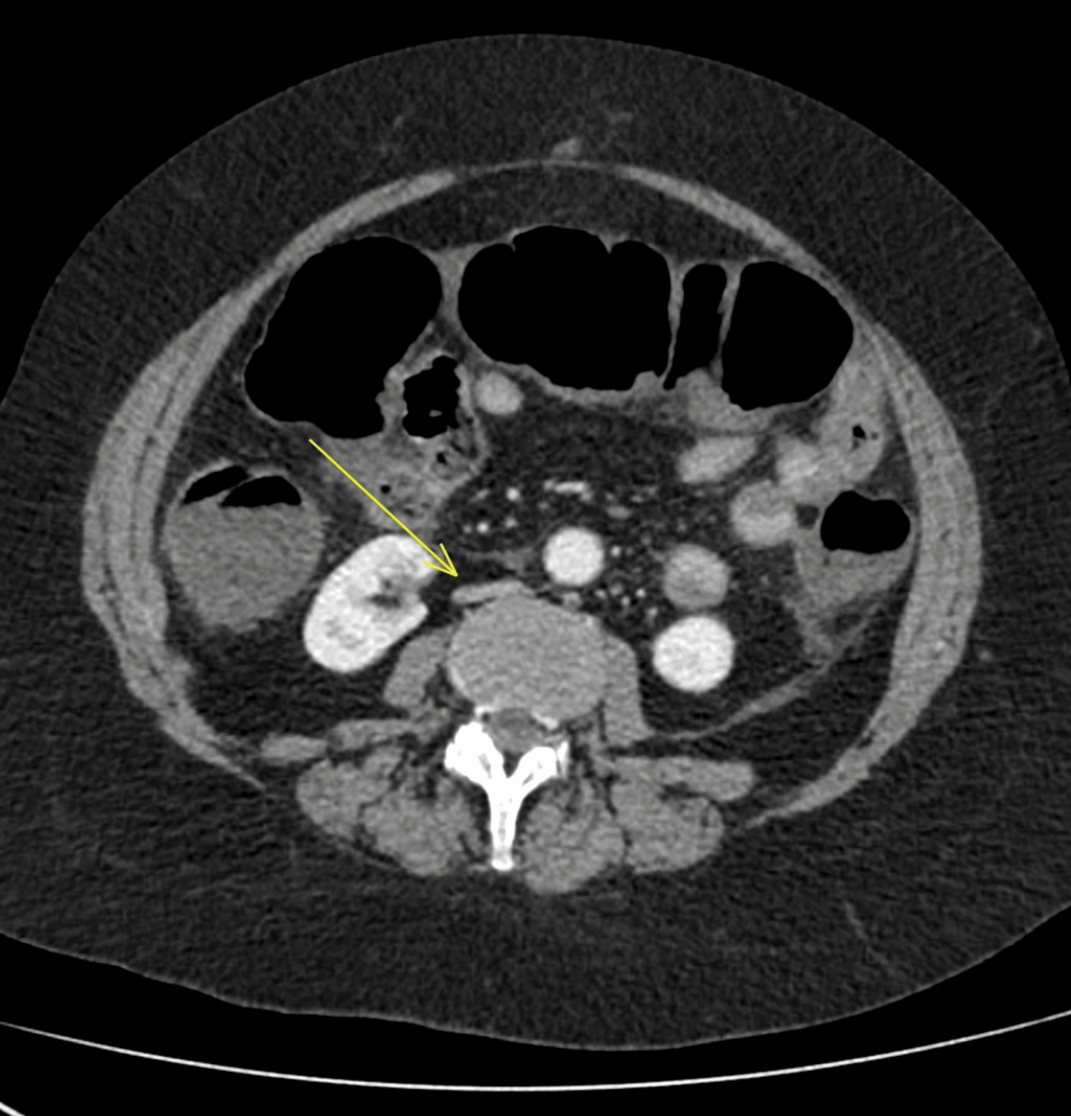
Axial view of abdominal CT showing IVC compression (arrow) and dilated large bowel loops CT: computed tomography, IVC: inferior vena cava
